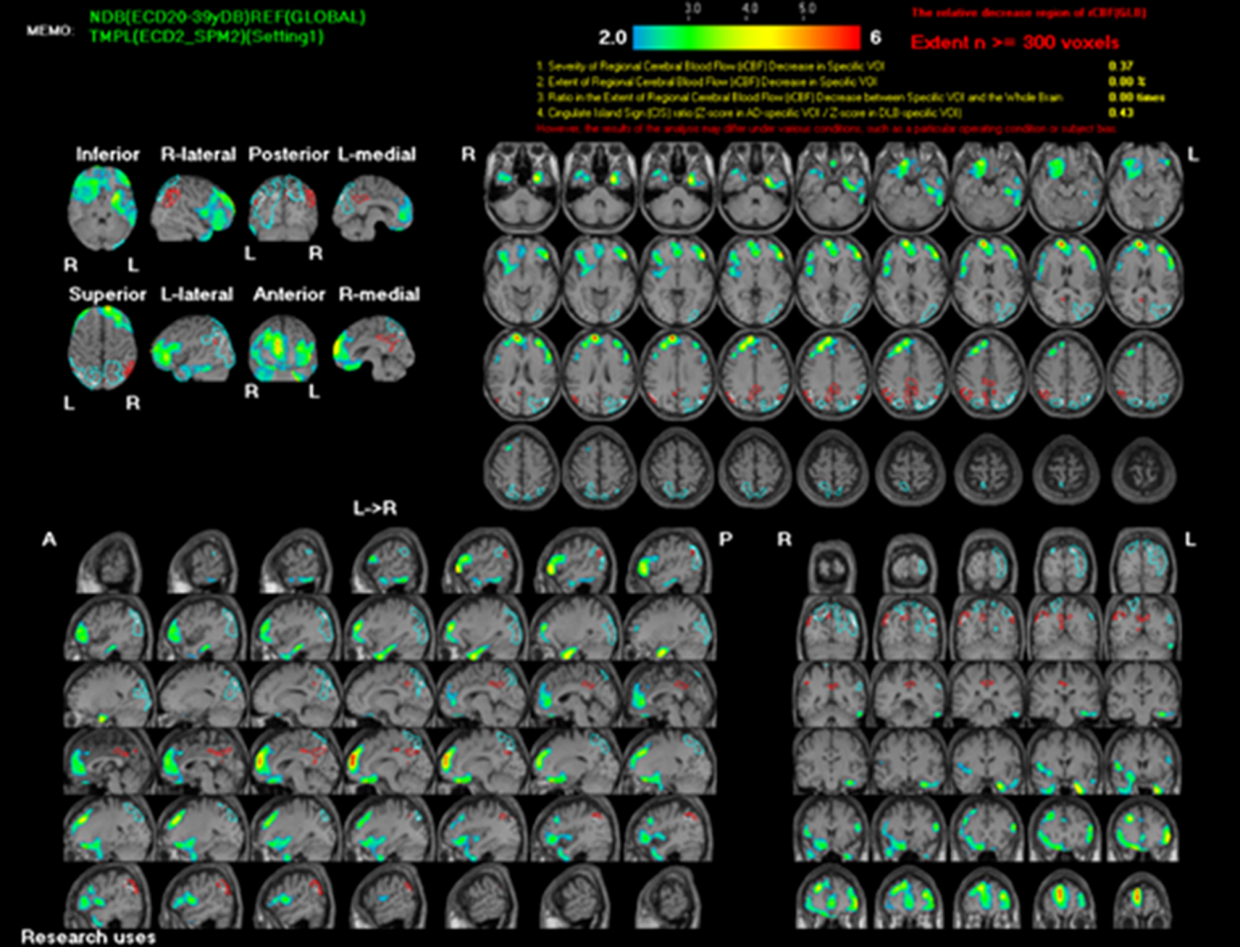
Tc-99m ECD brain SPECT scan shows mild to moderate hypoperfusion in bilateral frontal and left temporal regions.
